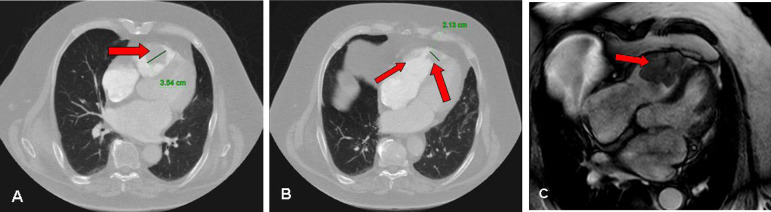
Radiological findings. (A, B) Contrast-enhanced axial computed tomography images through the heart showing tumor masses (red arrows) at the apex and anterior right ventricular wall of a measured diameter of 2.1-3.6 cm. (C) Magnetic resonance image obtained in long-axis projection through the right ventricle. The red arrow shows the mass filling the right ventricular cavity.
